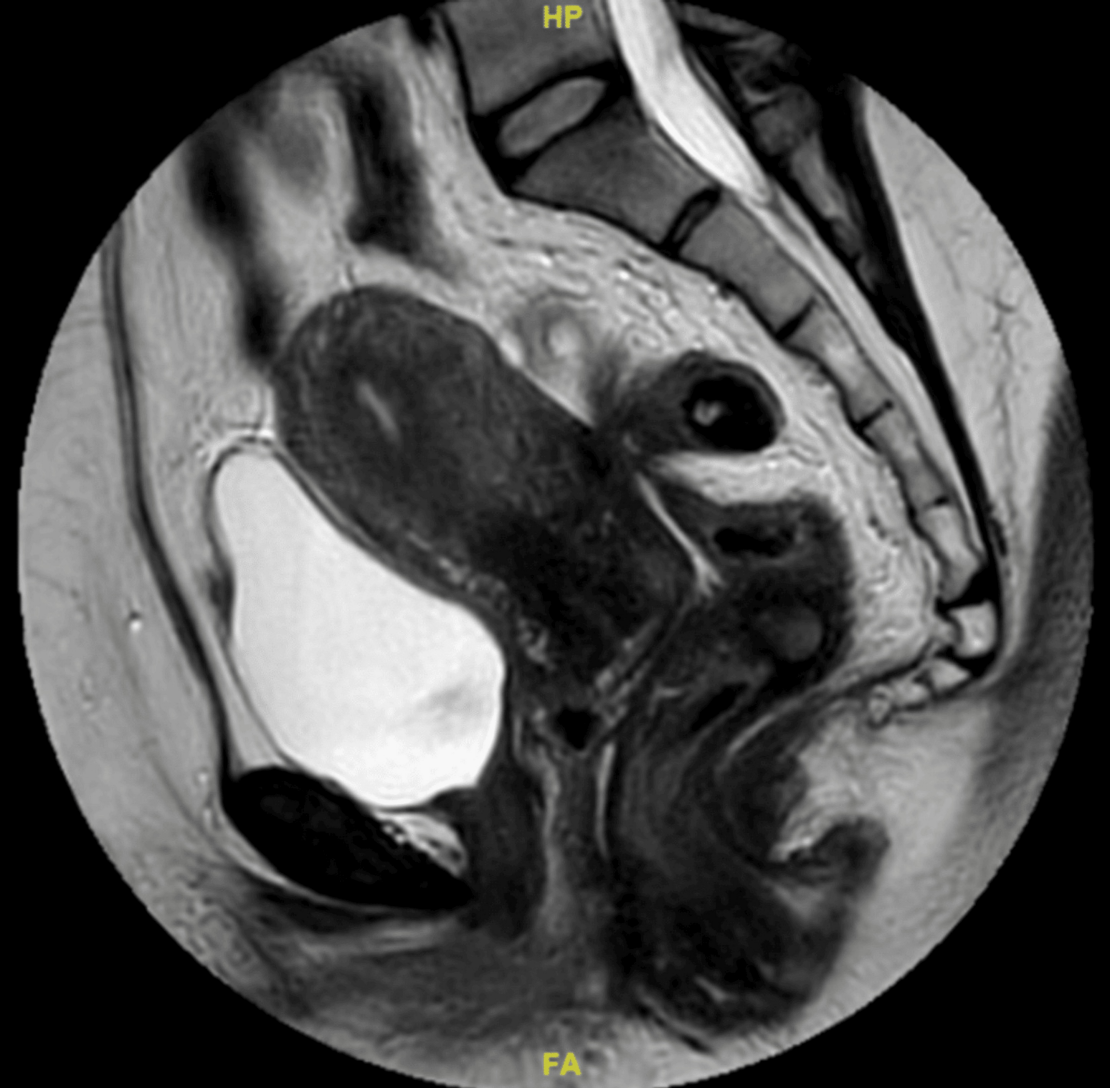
Sagittal magnetic resonance imaging of the pelvis following six doses of gonadotropin-releasing hormone analogue therapy, showing the marked regression of the rectal lesion.
